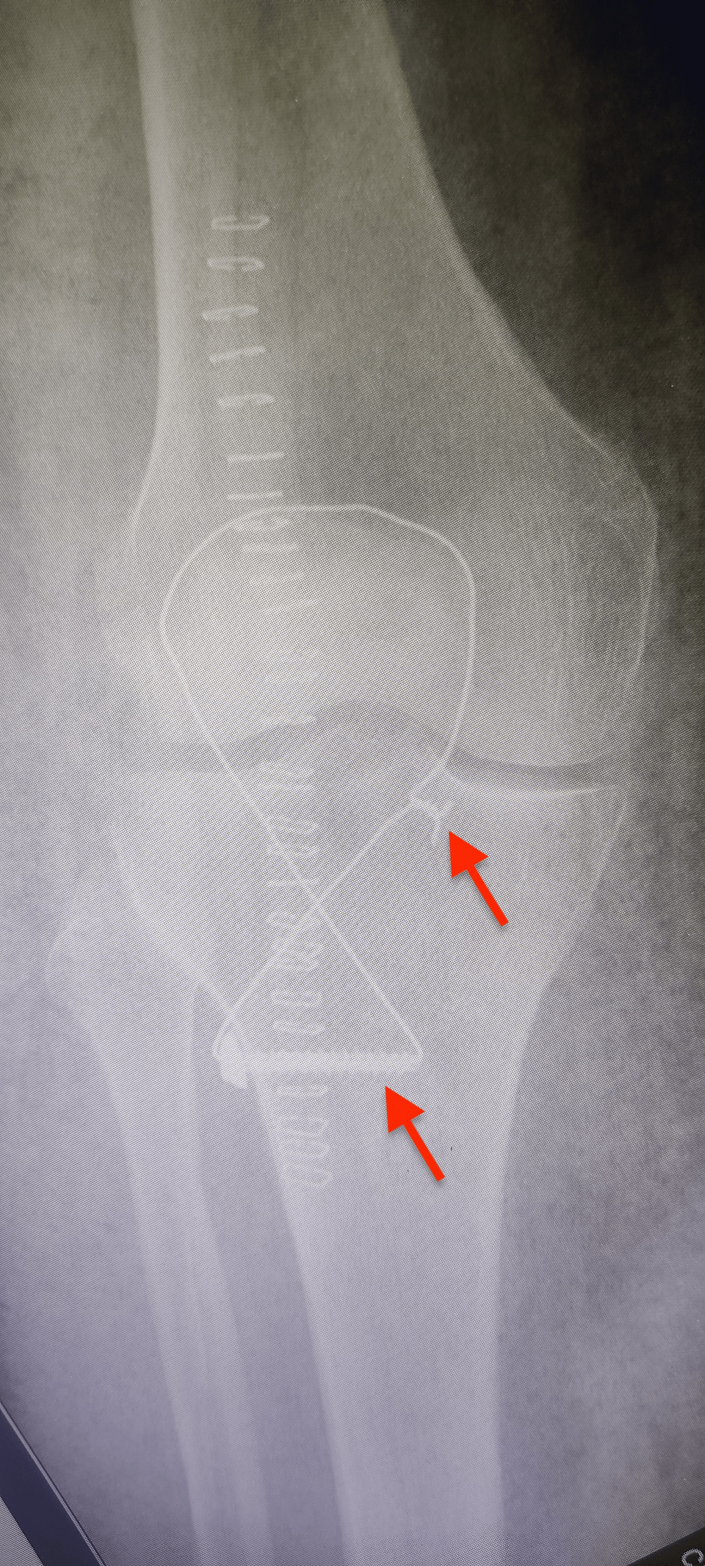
Postoperative anteroposterior X-ray of the left knee after partial patellectomy and fixation with tension band and tibial screw.
